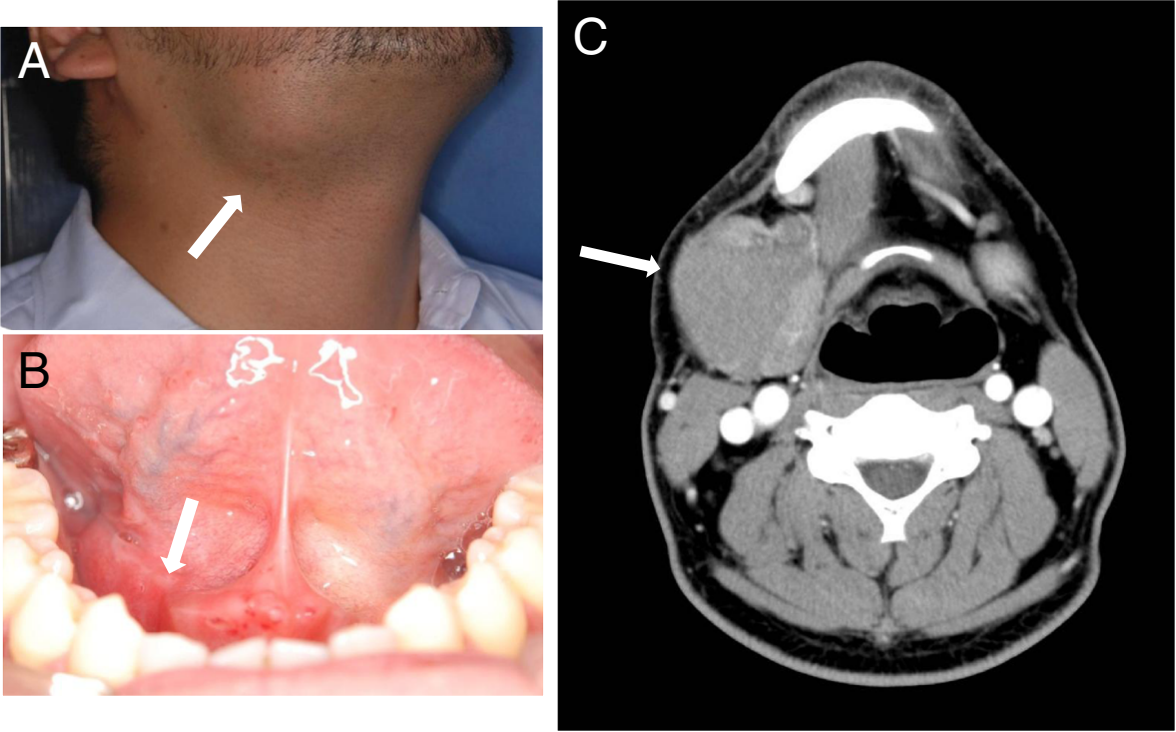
Clinical and computed tomography findings in the patient. (A) Right submandibular swelling. (B) Swelling in the right side of the floor of the mouth. (C) Computed tomography views revealing a cystic lesion involving the right submandibular gland.
